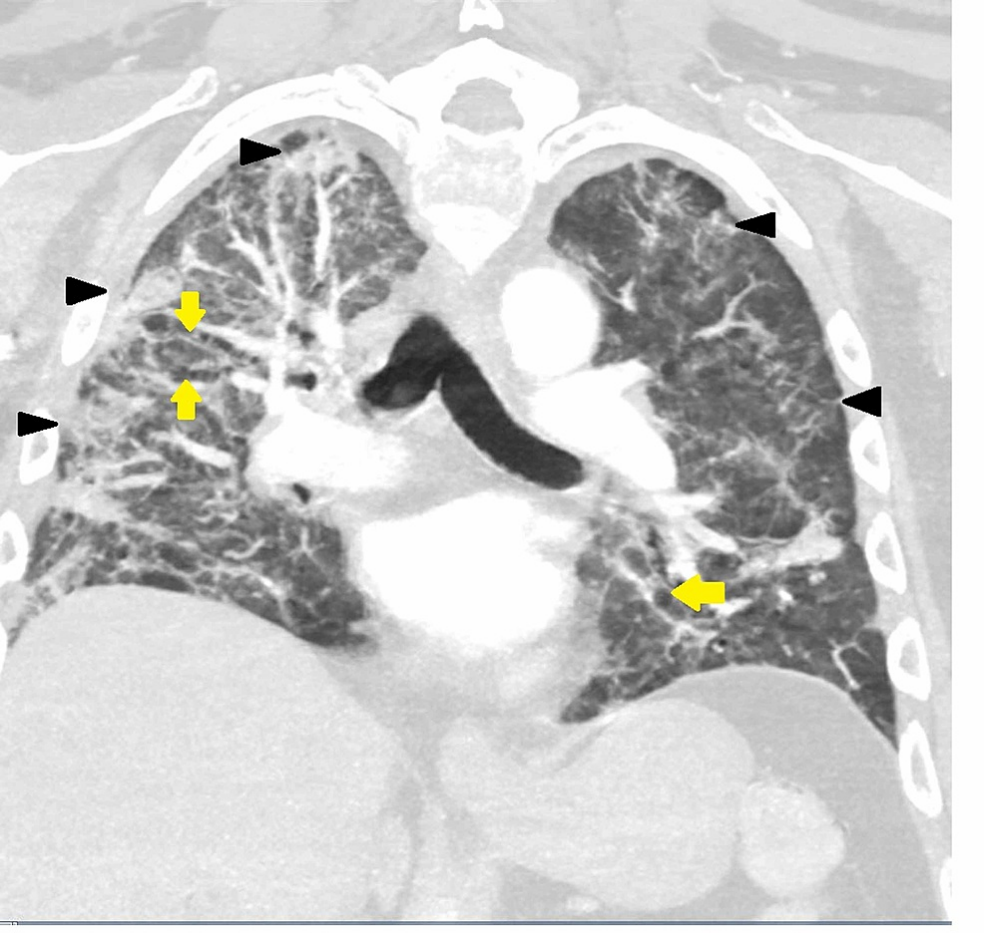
CT of the chest (coronal view) at week six: extensive diffuse bilateral pulmonary ground-glass opacities and air-space disease with architectural distortion bronchiectasis are seen. COVID-19 pneumonia/scarring is also noted. Black arrowheads show ground-glass opacities; yellow arrows show bronchiectasis
